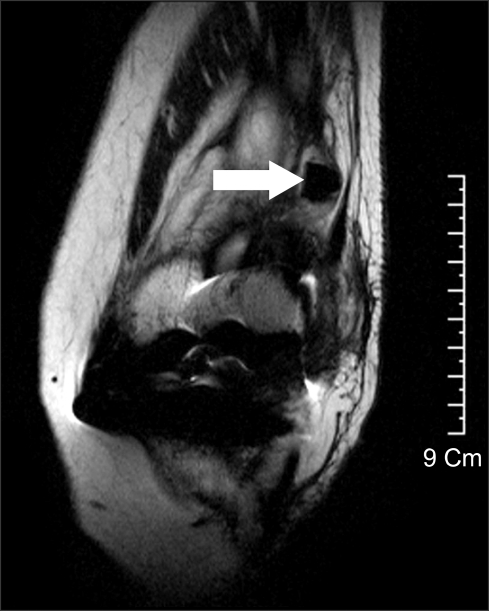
Coronal fast-spin-echo magnetic resonance image of the left knee in a patient following a slip and fall revealed a loose body in the suprapatellar pouch of the knee consistent in shape with the tip of the tibial polyethylene post.
